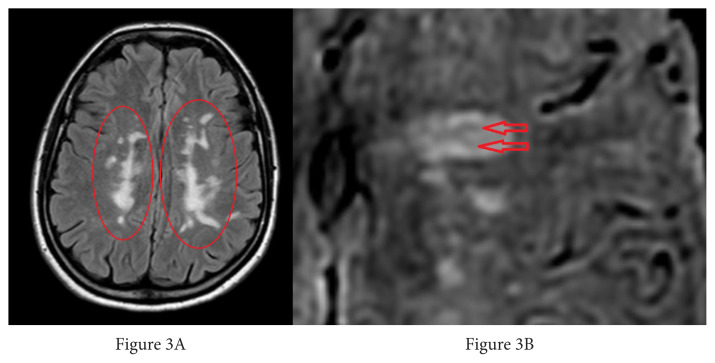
Examples of excluded lesions: A) White matter lesions with a bilateral tendency to merge were observed in the axial FLAIR image, and these lesions were excluded from the study. B) Axial SWI image, the lesion was not included in the study due to more than one hypointensity in the center of the periventricular white matter lesion located in the left frontal lobe.
